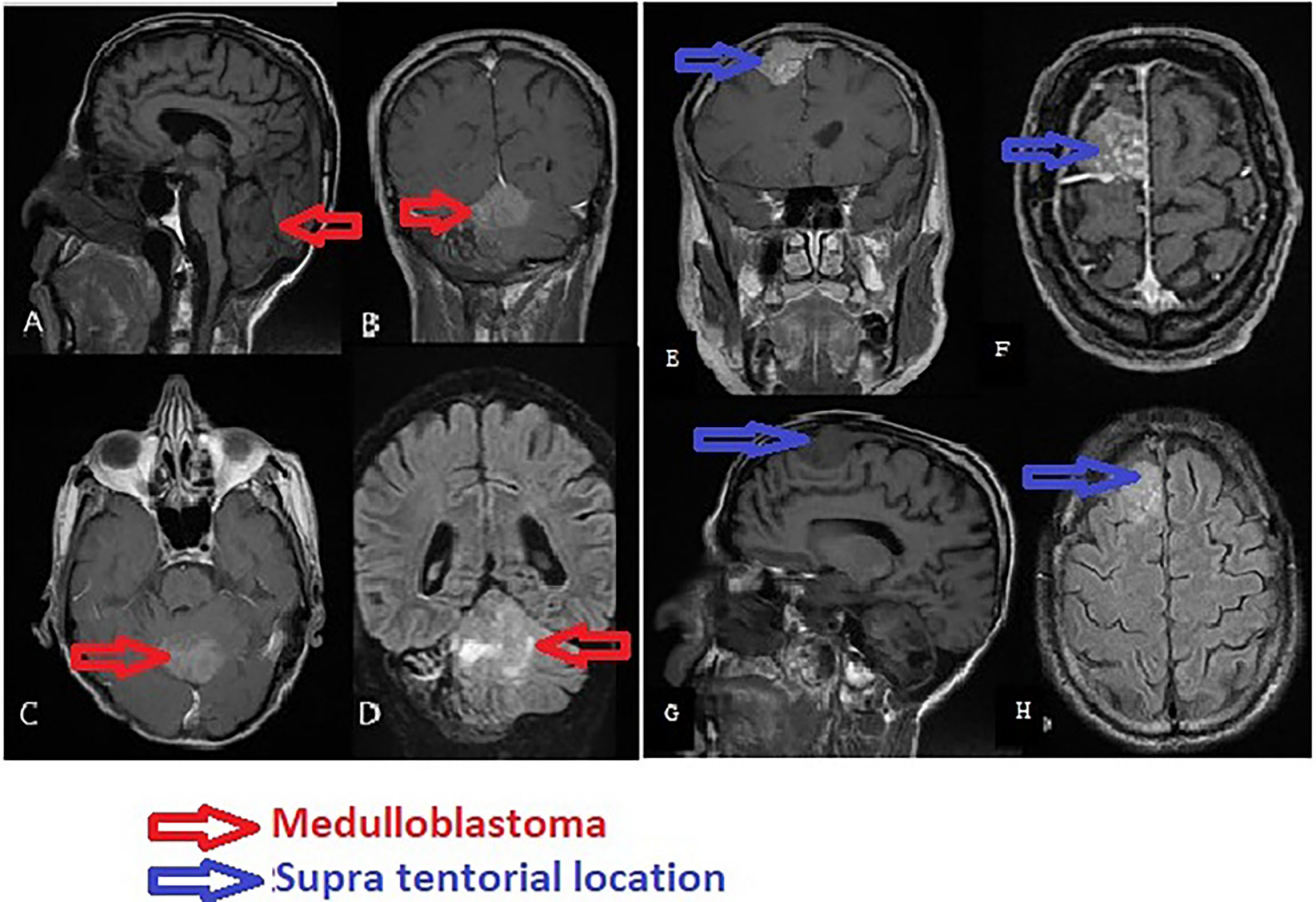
Magnetic resonance imaging of the brain showing glioblastoma of the posterior fossa and frontal meningioma. *1* Glioblastoma. (A) Sagittal T1. (B) Coronary T1-weighted post-gadolinium. (C) Axial T1 post-gadolinium. (D) Coronary fluid-attenuated inversion recovery (FLAIR). *2* Supratentorial location: probable meningioma. (E) Coronary T1 post-gadolinium. (F) Axial T1-weighted post-gadolinium. (G) Sagittal T1 plane showing the scalloping. (H) Axial FLAIR.
